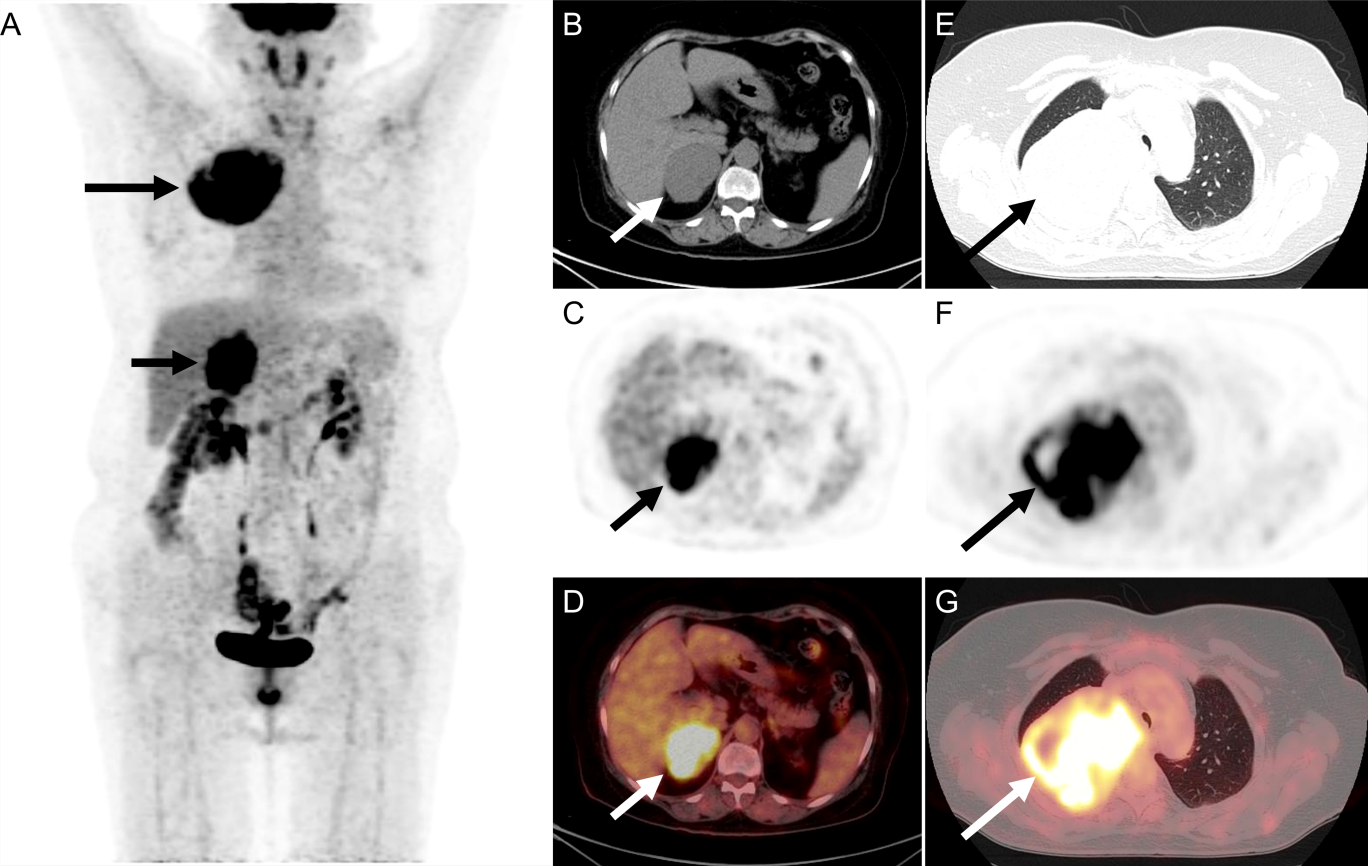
The ^18^F-FDG PET/CT of patient before operation. (A) The image revealed hypermetabolic lesions in the right thorax (long arrow) and the right upper abdomen (short arrow). (B–D) On the axial CT, PET, and fused PET/CT showed a large irregular lesion in the right lung upper lobe, measuring 8.4 cm×10.2 cm×8.1 cm showing intense ^18^F-FDG uptake with SUVmax of 13.4. (E–G) The axial CT, PET, and fused PET/CT revealed the increased activity corresponded to a slight hypodense right adrenal gland soft tissue mass, measuring 5.1 cm×6.3 cm×6.5 cm showing intense ^18^F-FDG uptake with SUVmax of 13.4.
